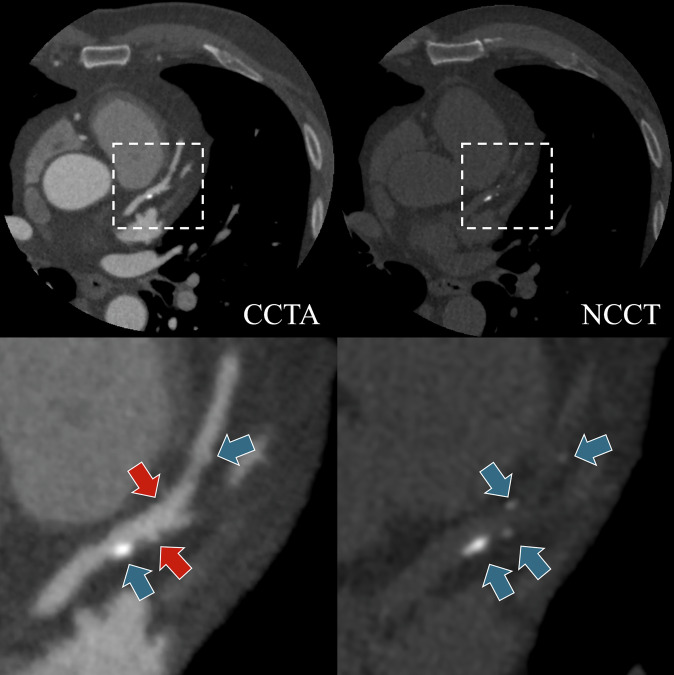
62-year-old male patient with calcified plaques missed by coronary CT angiography (CCTA) but detected by thin-slice non-contrast CT (NCCT). Thin-slice NCCT detected four distinct calcified plaques in the depicted segment of the left anterior descending artery (LAD). In CCTA, two of these plaques were missed (red arrows)
